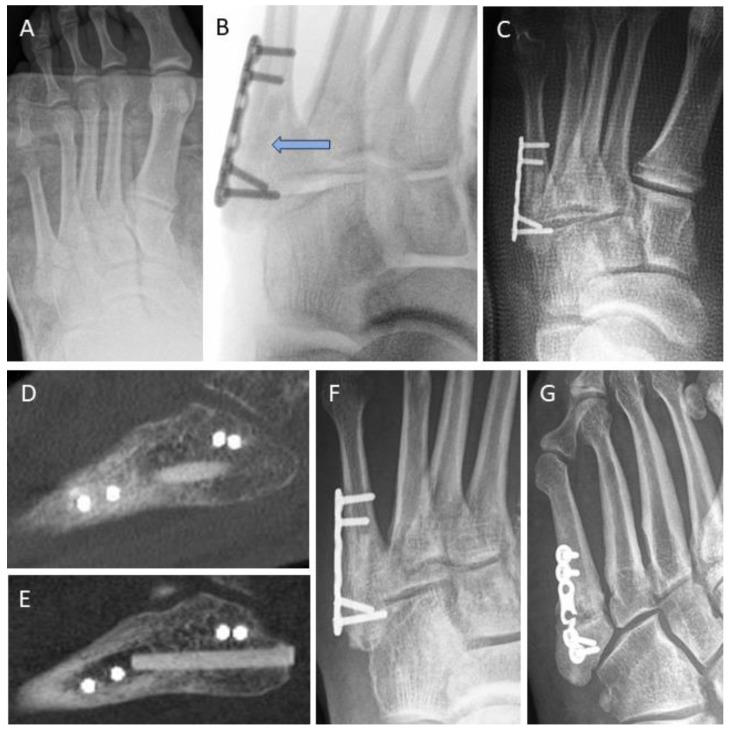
Non-union in a 36-year-old male professional handball player following an avulsion fracture at the base of the 5th metatarsal. (A) Conservative therapy that resulted in painful non-union. (B) Non-union surgery with a Shark Screw^®^ (blue arrow) and a metallic plate, additionally applied during the experimental phase in the surgeon’s learning curve with the allogeneic bone screw. (C) Post-operative radiograph 1 month after surgery. (D) CT scan 2 months after surgery. (E) CT scan 3 months after surgery showing an intact bone screw and bony union, allowing a return to professional handball. (F) Radiograph 4 months after surgery with an intact construct. (G) Remarkably, the plate experienced breakage after 5 months, without a refracture of the bone. The patient had only mild symptoms (skin irritation). Hardware removal was deemed necessary.
